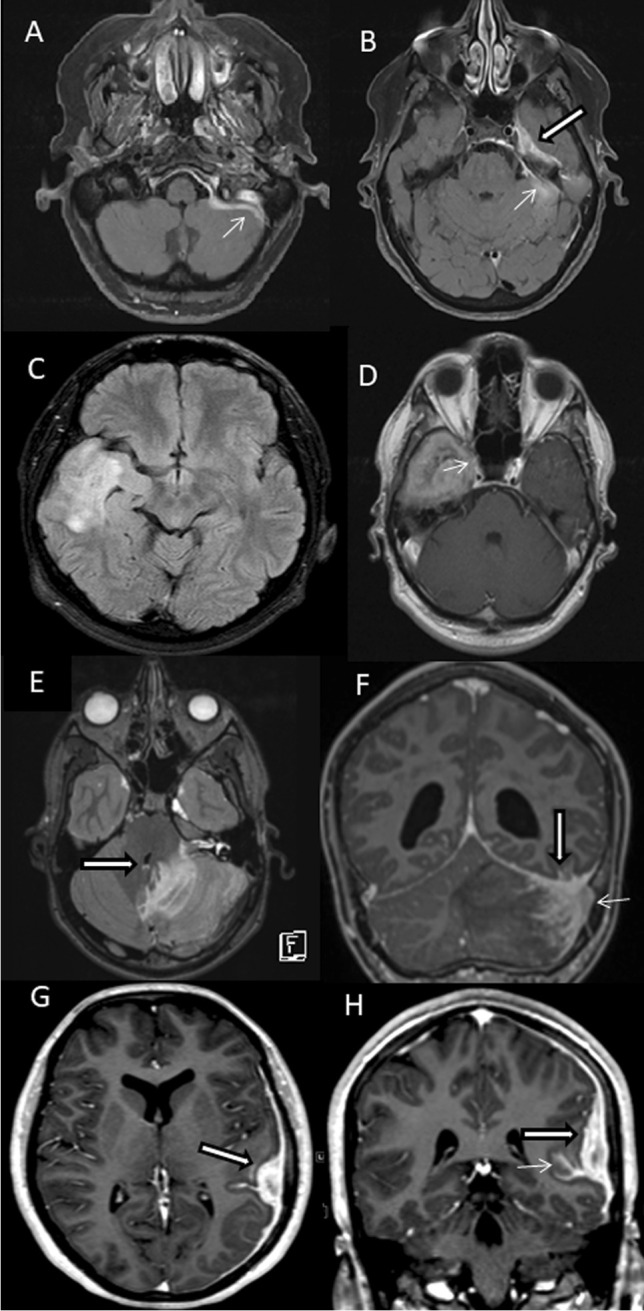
A, B Patient 1, 67-year-old woman: axial gadolinium (Gd)-enhanced fat-saturated T1-weighted MRI shows a heterogeneously contrast-enhancing extraaxial mass in the left middle fossa (large arrow) with infiltration of the dura extending to the posterior fossa (small arrows). C, D Patient 2, 30-year-old man: axial FLAIR-weighted MRI shows extensive cerebral edema in the middle and superior temporal gyrus. Axial Gd-enhanced T1-weighted MRI demonstrates homogenous contrast enhancing solid mass in the inferior temporal gyrus extending to the cavernous sinus (small arrow). E, F Patient 3, 16-year-old woman: T2-weighted axial images show extensive cerebral edema in the left cerebellar hemisphere with compression of the fourth ventricle (large arrow in E), and Gd-enhanced fat-saturated T1-weighted MRI shows heterogeneously contrast enhancing process infiltrating the cerebellar sulci, dural sinuses (small arrow), the left sided tentorium cerebelli and the ipsilateral sulci of the inferior temporal gyrus (large arrow in F). G, H Patient 4, 15-year-old man: T1-weighted gadolinium-enhanced axial and coronal MRI show a strongly contrast-enhancing left temporal tumor (large arrows) with finger-like extension to the adjacent sulci (small arrow)
